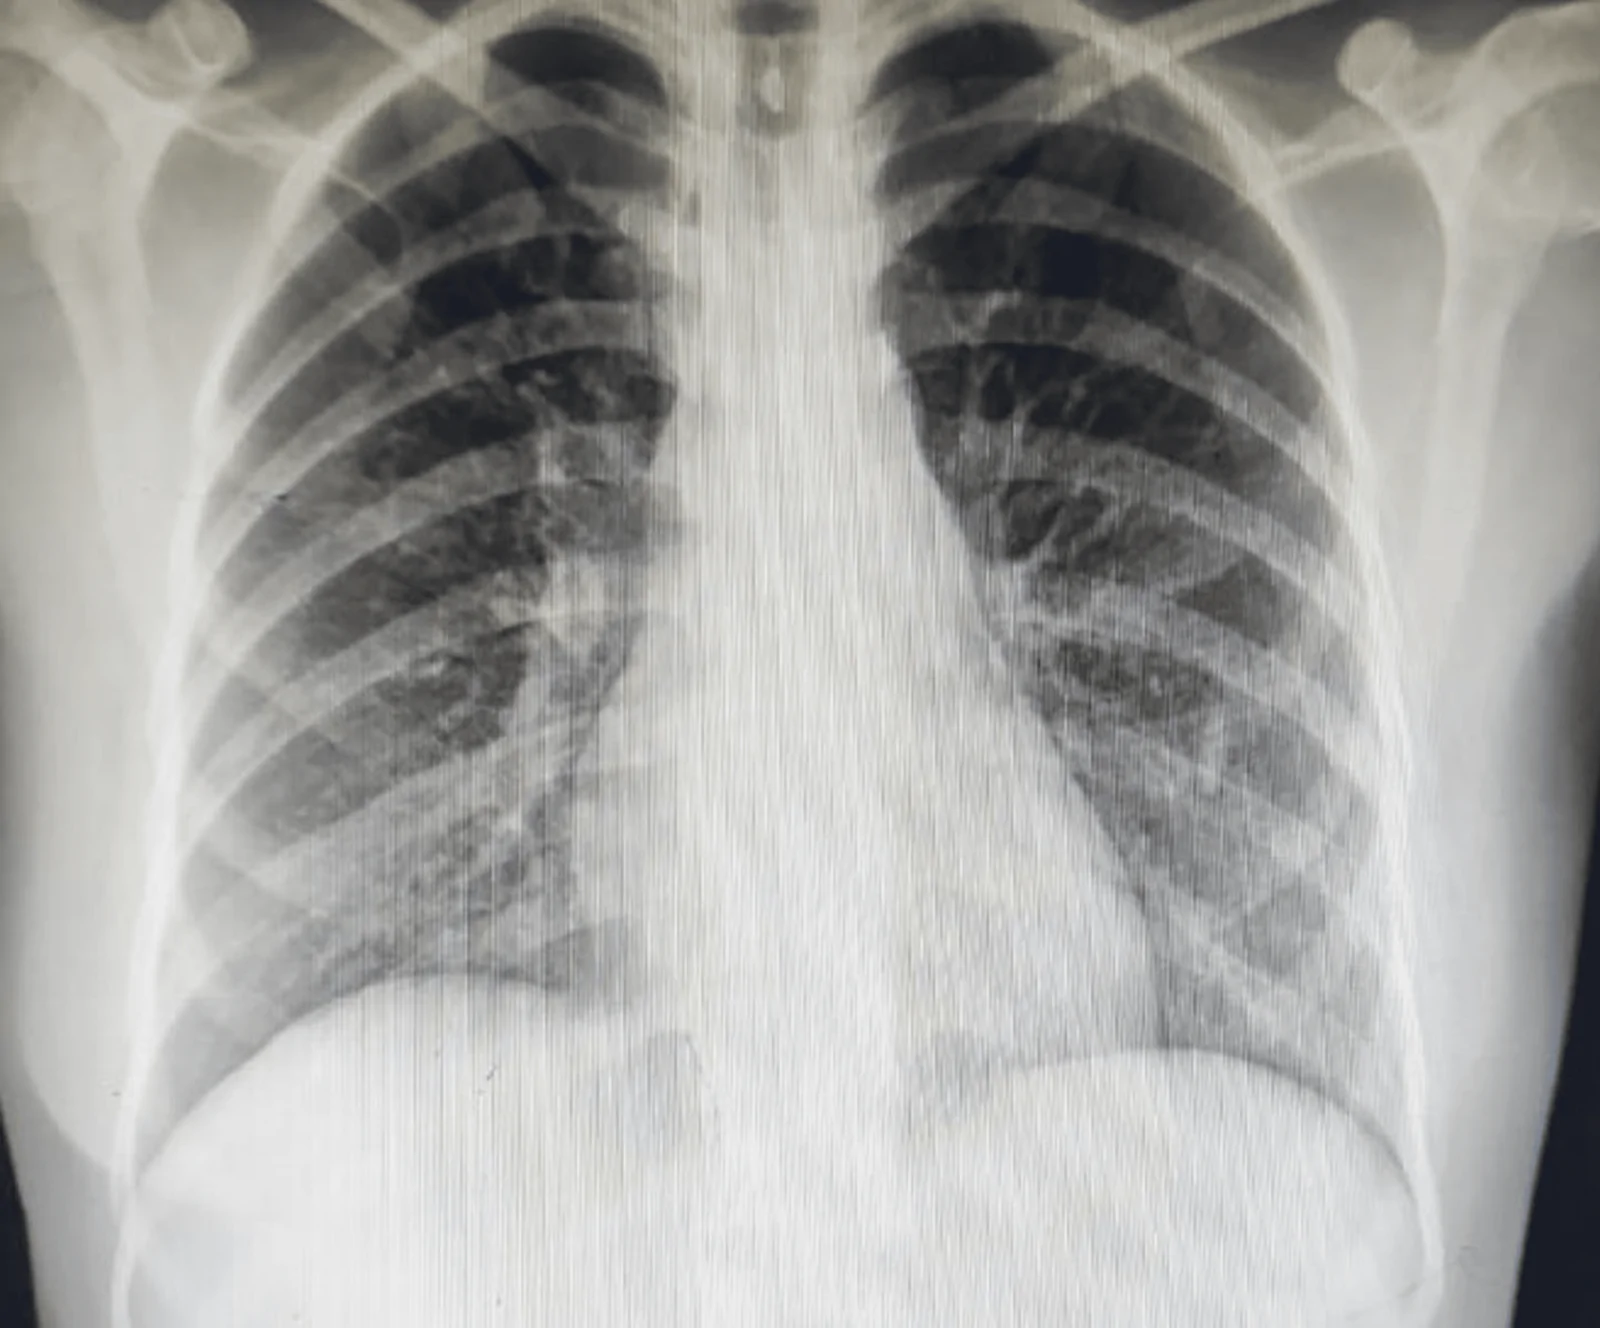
Chest X-ray of the patient
